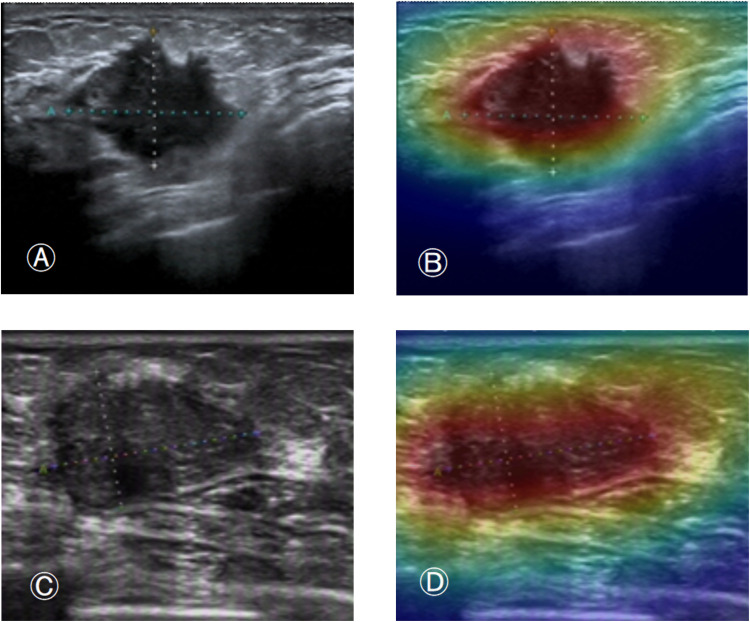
Figures A and C show the B-mode ultrasound images of invasive ductal carcinoma and mucinous carcinoma in the test set, respectively, and Figures C and D show the respective images after overlaying the thermograms using the pre-trained DenseNet121 model.
